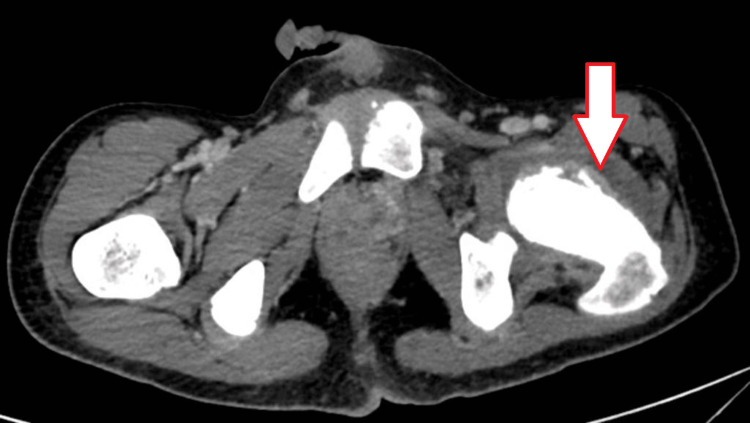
CT pelvic scan showing the presence of a suspicious lesion involving the left femoral neck
